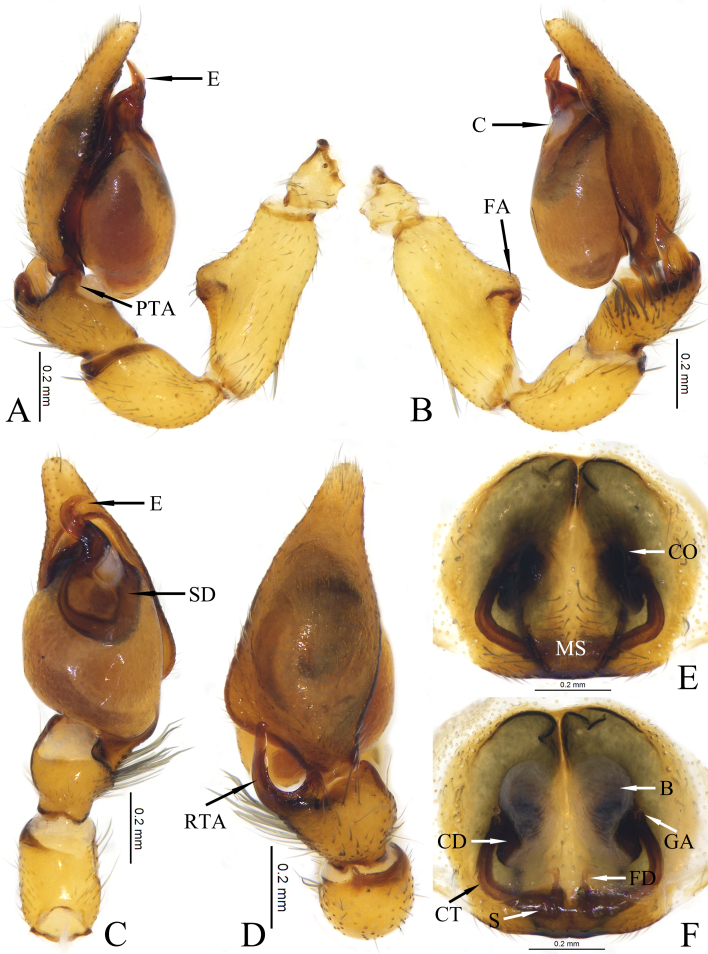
Copulatory organs of *Otaciliasubshanxi* sp. nov.: A Male left palp, prolateral view; B Same, retrolateral view; C Same, ventral view; D Same, dorsal view; E Epigyne, ventral view; F Same, dorsal view. Abbreviations: E—embolus; FA—femoral apophysis; PTA—prolateral tibial apophysis; RTA—retrolateral tibial apophysis; SD—sperm duct; B—bursa; CO—copulatory opening; CD—copulatory duct; CT—connecting tube; FD—fertilisation duct; GA—glandular appendage; MS—median septum; S—spermathecae.
